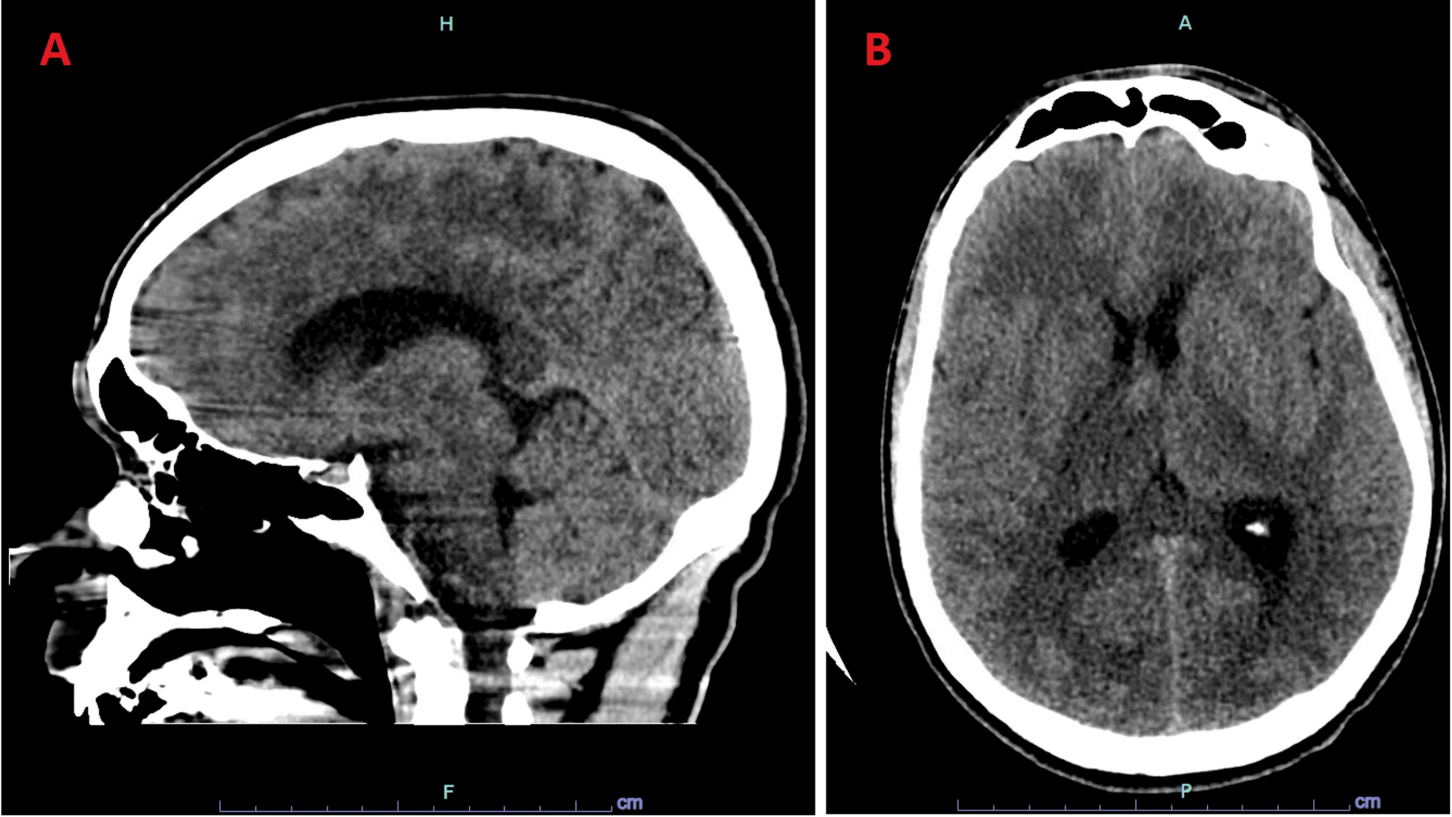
Sagittal and axial non-contrast CT of the head at presentation. Sagittal (A) and axial (B) views of the initial CT imaging which was negative for acute hemorrhage but demonstrated questionable patchy decreased attenuation in the bilateral parietal lobes and right thalamus. CT: computed tomography
